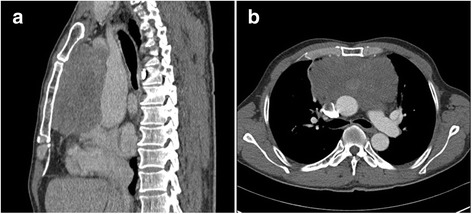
Chest computed tomography (CT). a, b The tumour mass was located in the anterior mediastinum with heterogeneous enhancement
